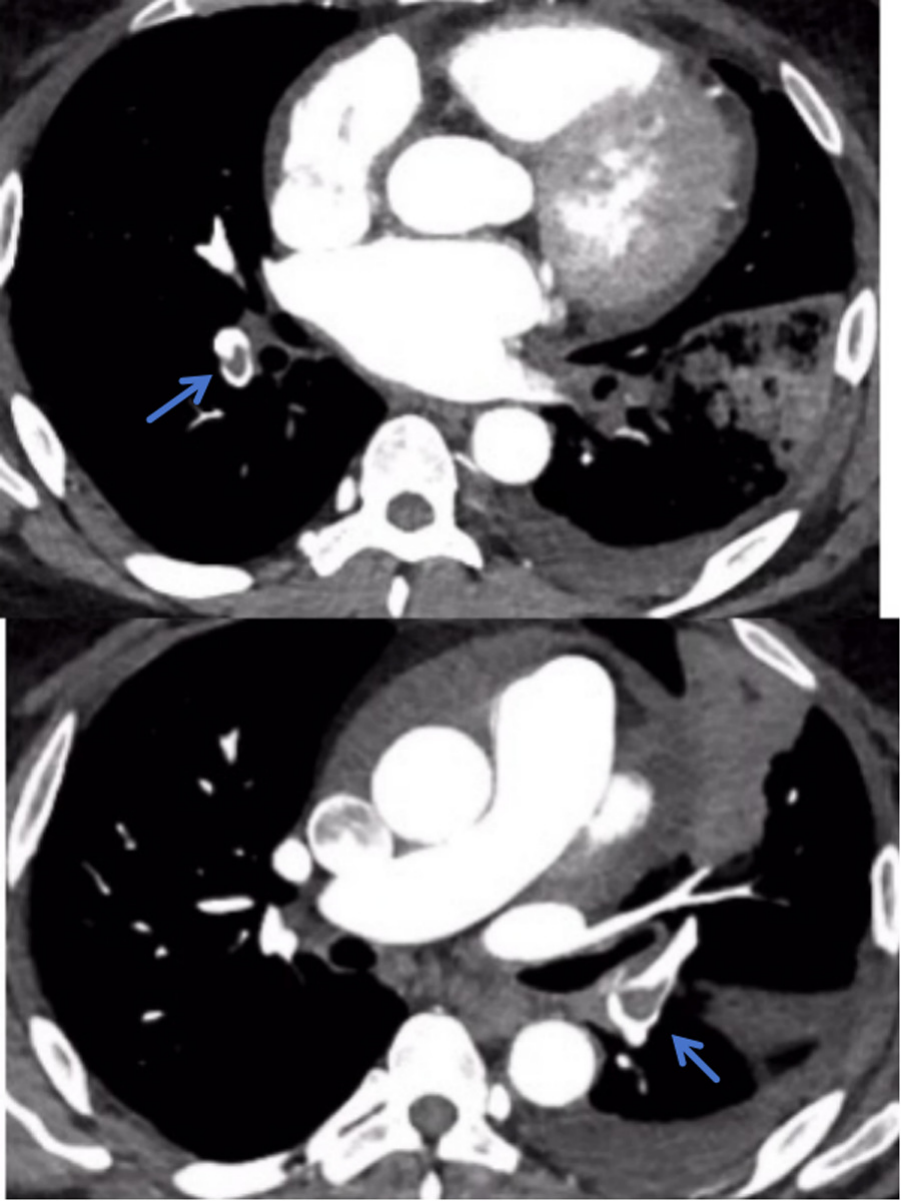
TRO CTA showed bilateral lower pulmonary artery/branch thrombi.
